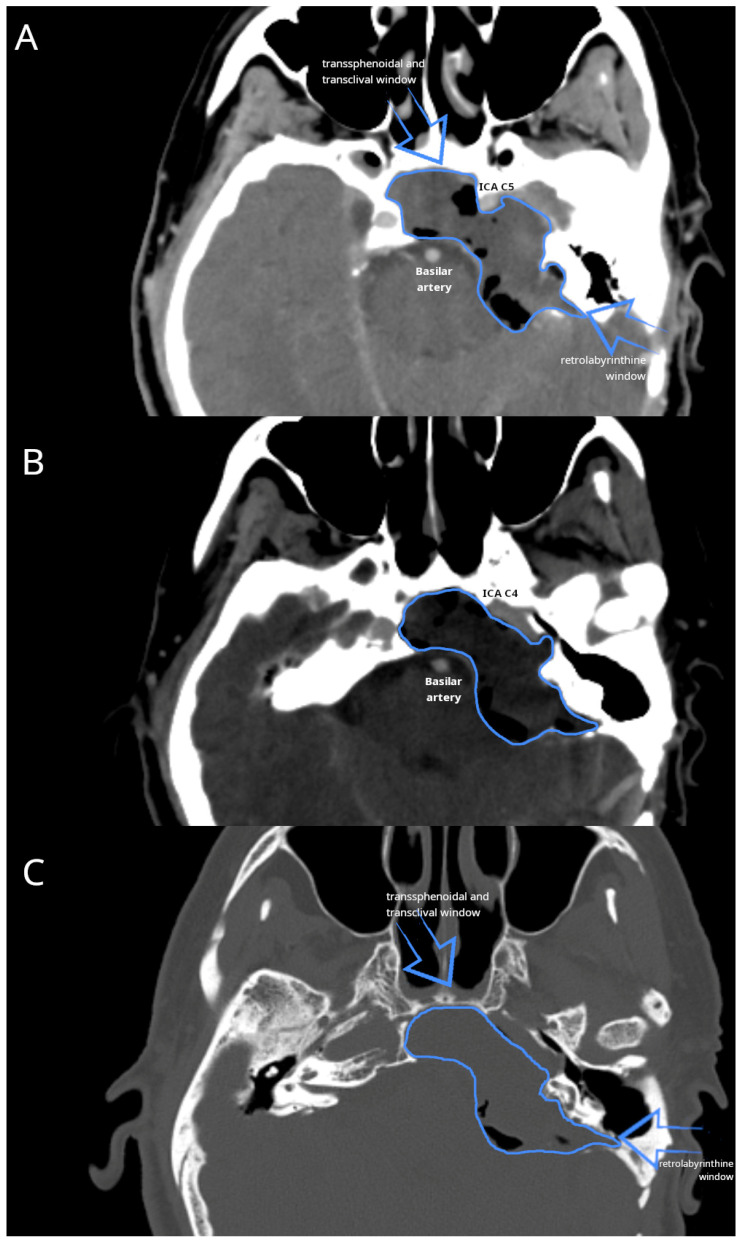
Preoperative CT imaging of the lesion. ICA (internal carotid artery), basilar artery, and cholesteatoma (encircled in blue) are indicated. (A,B) CT angiography; (C) high-resolution CT image.
